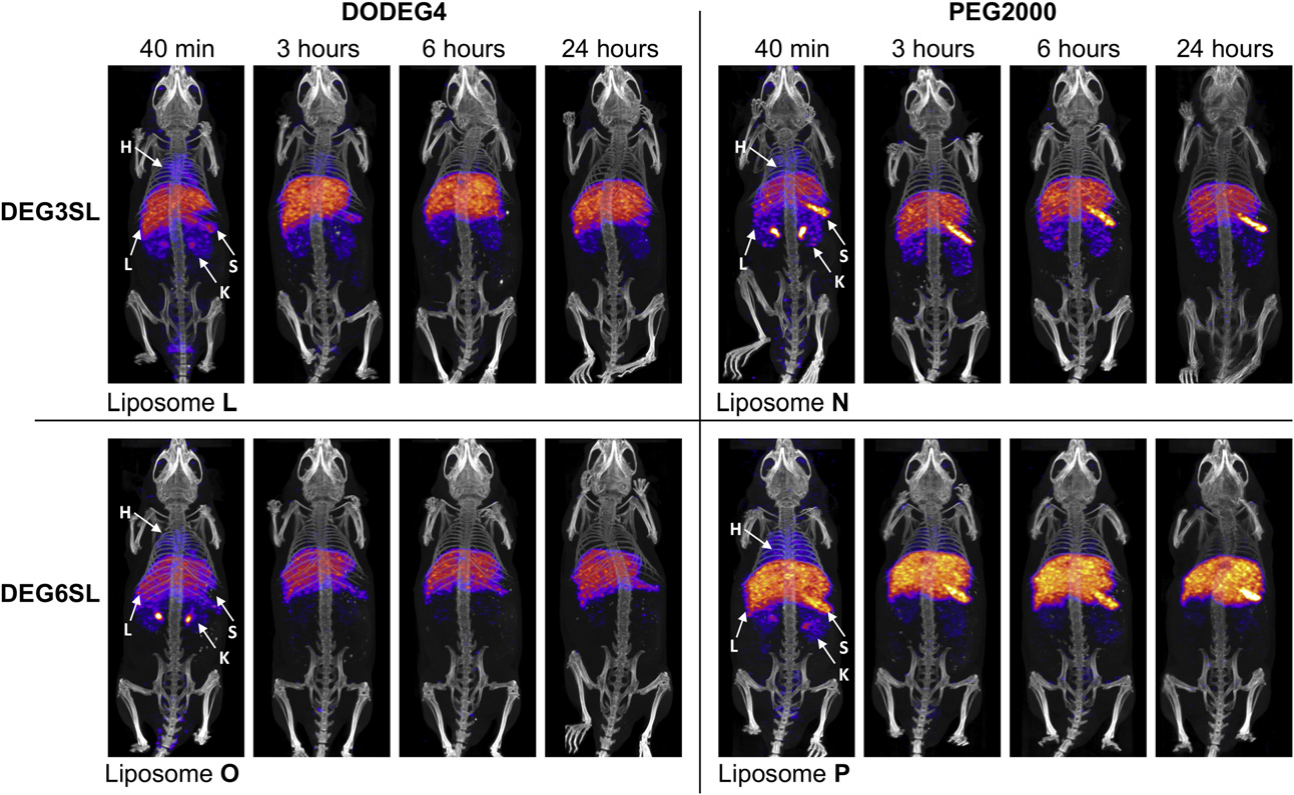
In vivo SPECT/CT distributions derived from an intravenous administration of ∼10MBq labelled DODEG4 (left hand column) liposome preparations L and O, formulated with DEG3SL and DEG6SL respectively, compared to the control DSPE-PEG2000 (right hand column) liposome preparations N and P formulated with DEG3SL and DEG6SL respectively, over the course of 24 h (H – heart, L – liver, S – spleen, and K – kidney). The bladder has been removed from the 40 min and 3 h images, allowing the images to be scaled so they were comparable to each other.
